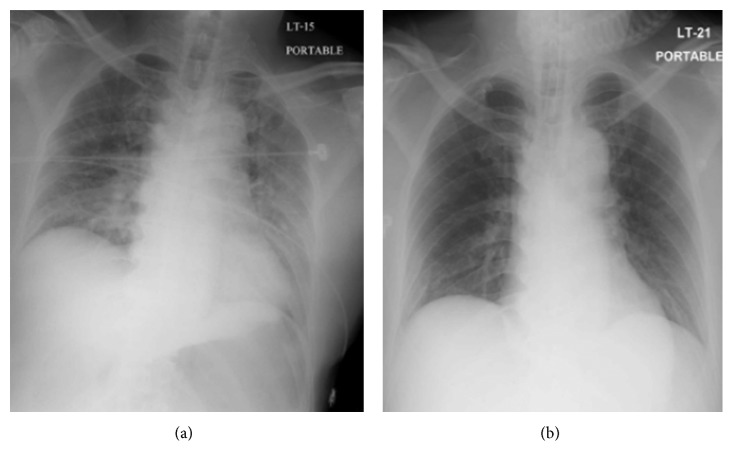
Chest radiograph at 1 hour after surgery (a) and 24 hours after surgery (b).
